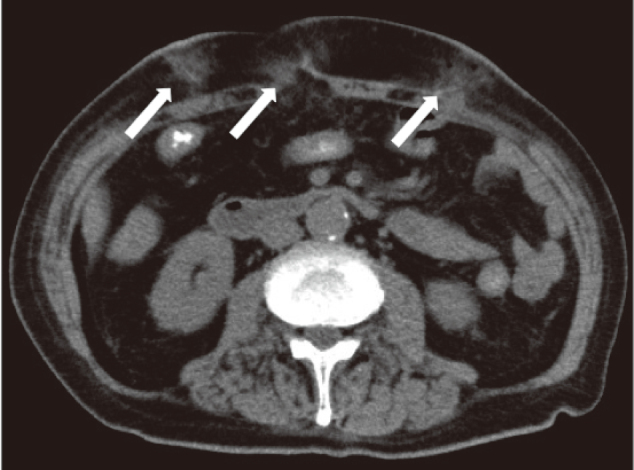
Computed tomography findings No postoperative intra-abdominal events but fluid retention in the subcutaneous tissue of the port site.
